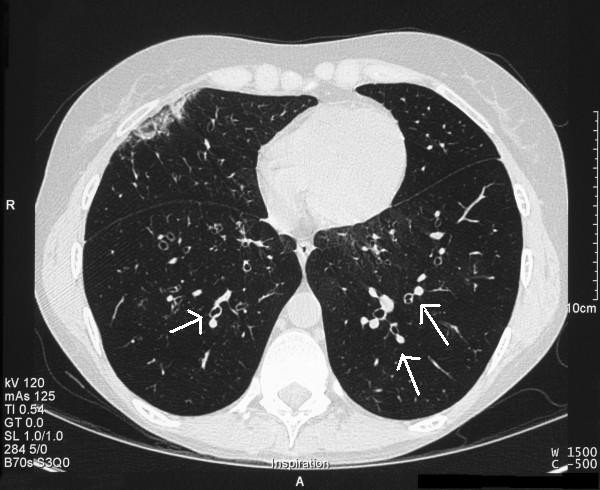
October 2005 (CT Thorax): Resolution of areas of consolidation and "signet ring sign". The signet ring sign is a finding seen on CT scans of the thorax. It consists of a small circle of soft tissue attenuation that abuts a ring of soft tissue attenuation surrounding a larger low attenuating circle of air and is indicative of bronchiectasis.
